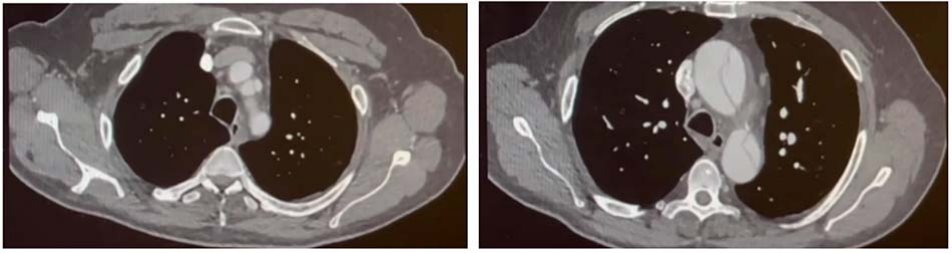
Follow-up CT aortogram. Aortic dissection extending into the left common carotid artery and involving the left subclavian artery without compromising the lumen. The ascending aorta has further dilated from Fig. 1 to now measure ~51 mm.
